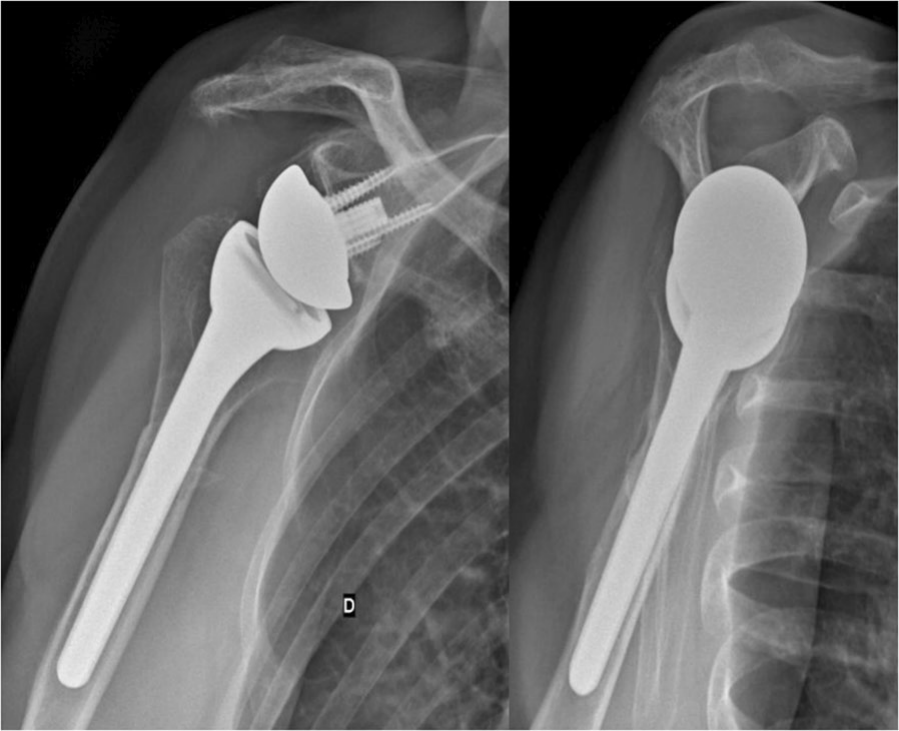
Radiograph of a right shoulder reverse shoulder arthroplasty with concomitant latissimus dorsi transfer with osteolysis at the latissimus dorsi tendon insertion site
